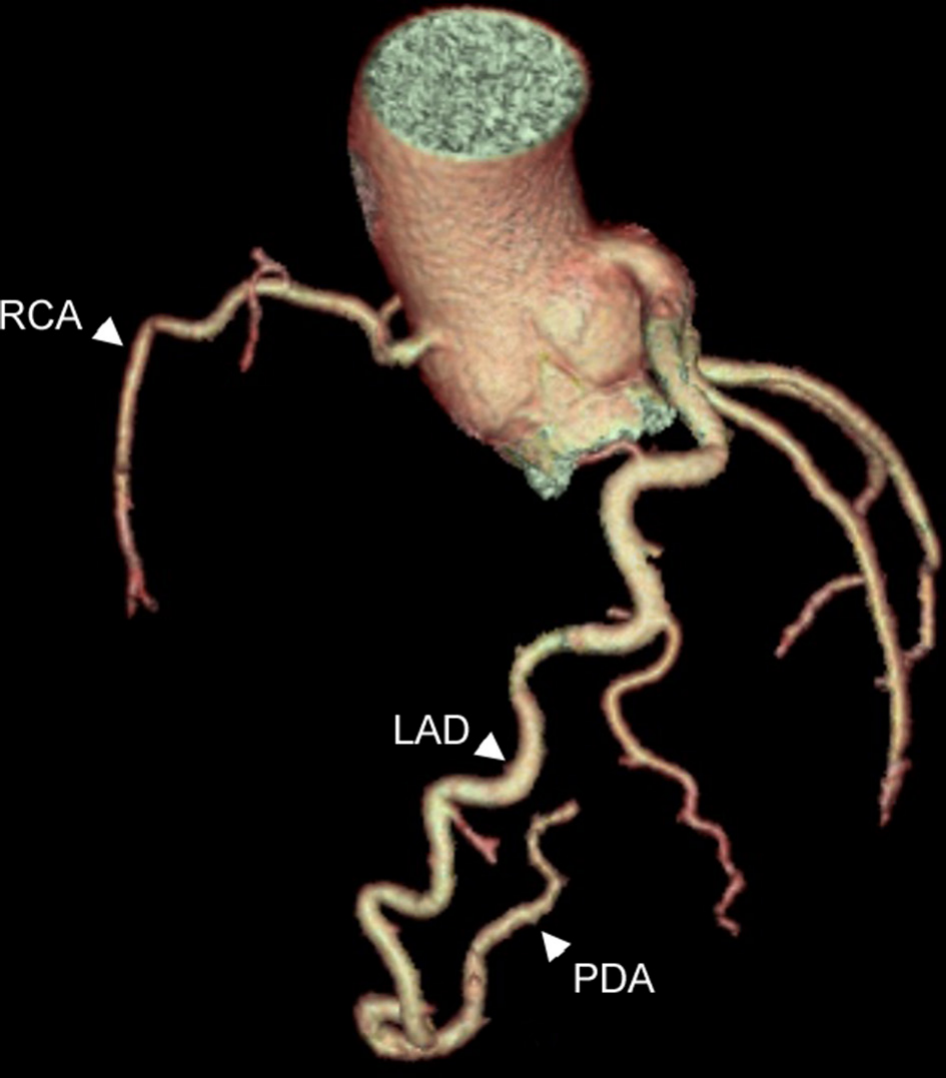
3D-reformat of CT coronary angiogram Figure showing a very tortuous and large calibre left anterior descending artery (LAD) located in its normal territory, but with the anomalous posterior descending artery (PDA) seen arising from it distally. The right coronary artery (RCA) is small and non-dominant.
